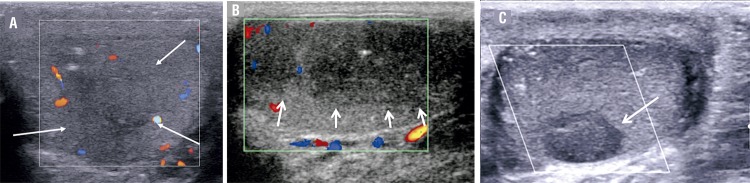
Testicular Color Doppler Ultrasound of three patients (in the same order) demonstrating avascular hypoechoic areas (white arrows) with some microlithiasis suggestive of burned out tumors.
